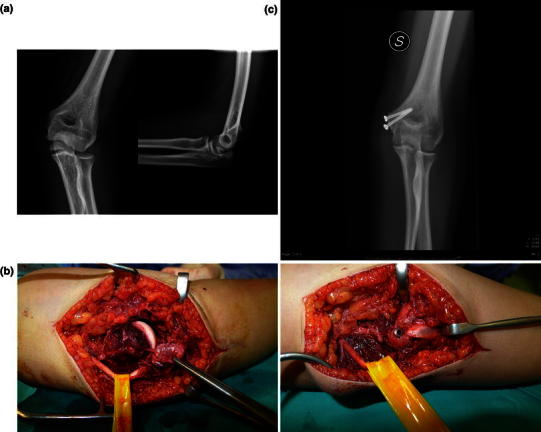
Case 1.a X-ray showing medial epicondyle fracture with intra-articular fragment incarceration.b Intraoperative view of ulnar nerve identification and protection followed by open reduction and internal fixation with two cannulated screws.c X-ray at 3 months, showing complete healing of the fracture
